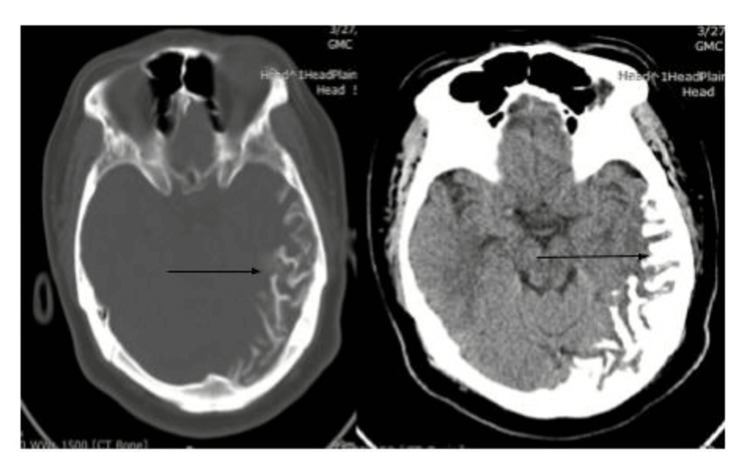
CT (brain Window) showing curvilinear leptomeningeal calcification is seen along left temporoparietal sulcal spaces as seen by the arrow CT: computed tomography
